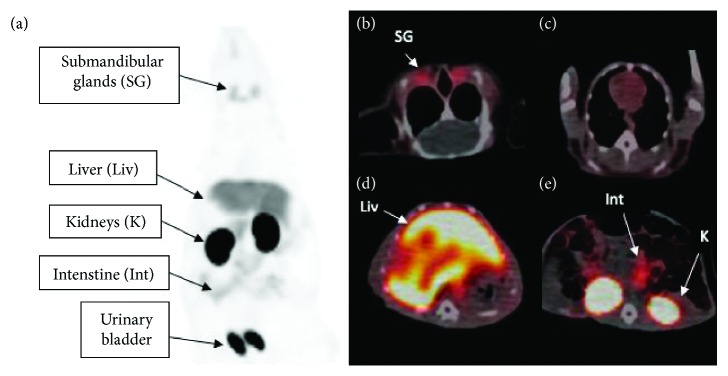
Whole-body [^11^C]-metformin PET scans were performed after i.v. tracer injection. (a) Attenuation corrected maximum intensity projection of [^11^C]-metformin biodistribution in a chinchilla 30 min after injection demonstrates uptake in submandibular glands, liver, kidneys, intestine, and urinary bladder (indicated by arrows). Transaxial slices of [^11^C]-metformin biodistribution on merged PET/CT images (arrows indicate metformin avid organs) are displayed in (b) submandibular glands, (c) thorax, (d) liver, and (e) kidneys.
